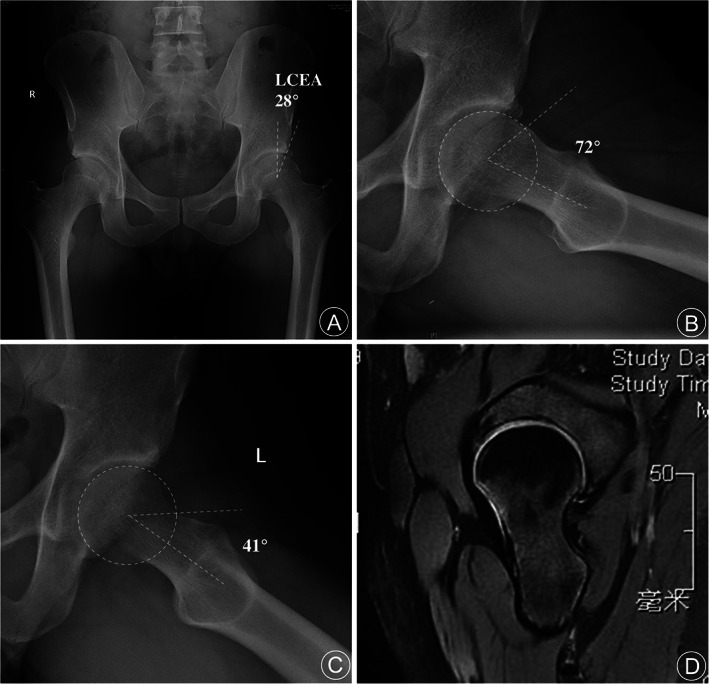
The radiologic outcome of a 32‐year‐old male patient with cam FAI underwent hip arthroscopy with longitudinal outside‐in capsulotomy. (A) The preoperative anteroposterior pelvic view with normal acetabular coverage (LCEA 28°) and os acetabuli on the left hip. (B) The preoperative Dunn view of the left hip with a significant bump of cam lesion in head–neck junction (α angle 72°). (C) The postoperative Dunn view of the left hip with the normal off‐set of head–neck junction established (α angle 41°). (D) The hip MRI image at 1‐year follow‐up shows that the capsule completely healed, and there is no adhesion and effusion.
